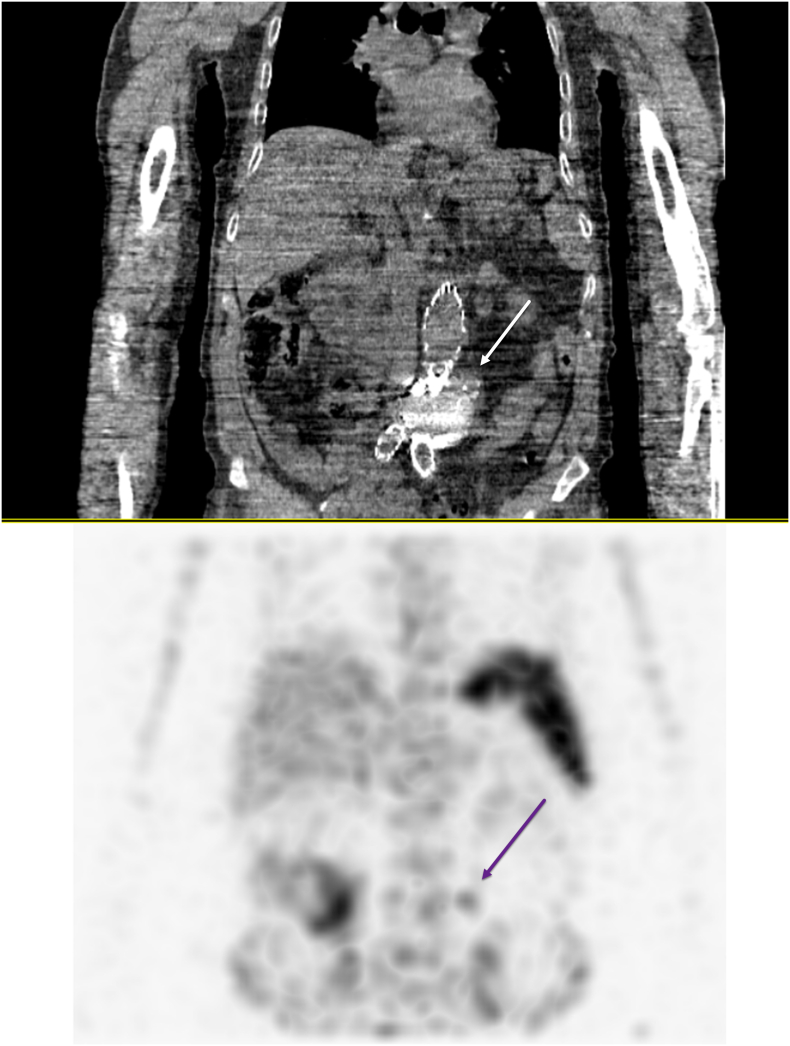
Planar images were taken at 60 min, 4 to 6 h and 24 h post-injection of 285 MBq of ^99m^Tc-HMPAO-labelled white blood cells respectively. The accumulation of white blood cells in two foci was consistent with two septic foci. The SPECT/CT images at 4 to 6 h post-injection confirmed the presence of two foci of white blood cell accumulation in the distal part of the prosthesis.
